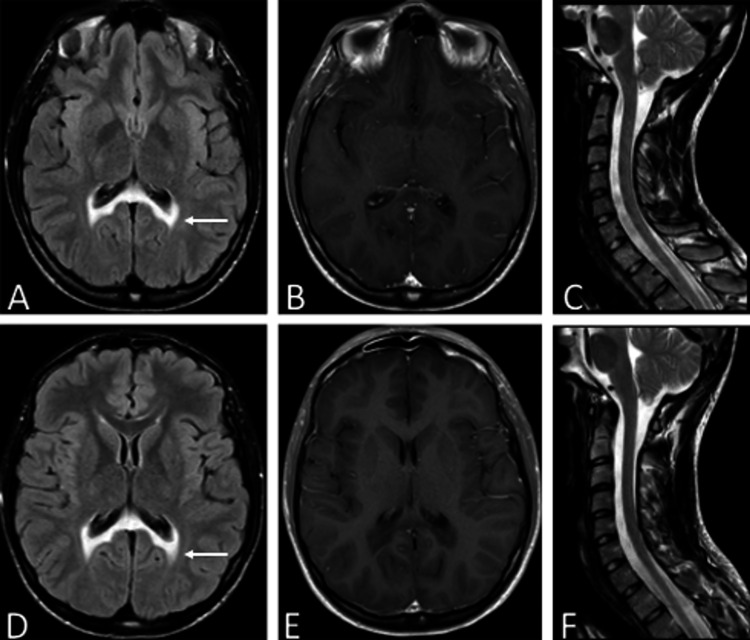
Imaging similarities in the brain and cervical spine of the twin patients. Brain MRI, axial T2 fluid-attenuated inversion recovery (A) and (D) and axial T1 post-gadolinium (B) and (E), demonstrating T2 hyperintensity in the splenium of the corpus callosum and the parieto-occipital deep white matter (white arrow: (A) and (D)) and no abnormal enhancement (B) and (E). Cervical spine MRI, sagittal T2 sequences demonstrating similar normal tissue signal and cord caliber (C) and (F). Patient A (A)-(C) and patient B (D)-(F).
